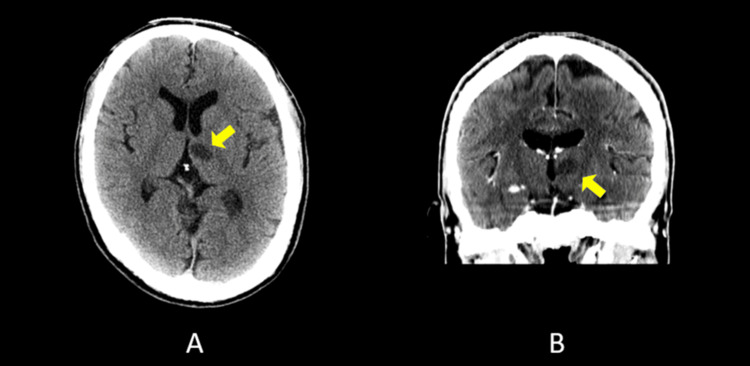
Non-contrast head CT obtained at admission showing a left anterior thalamic infarction Non-contrast head CT images obtained at admission demonstrate a focal low-density lesion in the left anterior thalamus (yellow arrows). Panel (A) shows an axial slice, and Panel (B) shows a coronal slice. No cortical lesions are observed. CT: computed tomography
